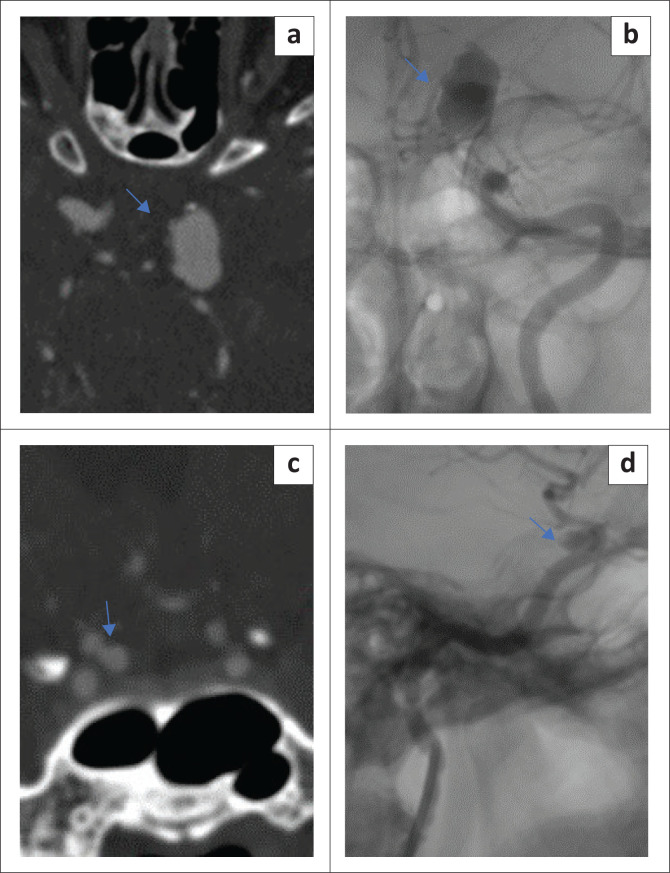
(a) CT angiography axial and (b) left internal carotid artery angiogram (non-subtracted image) frontal view showing a medium-sized (13 mm) fusiform aneurysm arising from the supraclinoid segment of left internal carotid artery (arrows) in a 65-year-old female. (c) CT angiography coronal maximum intensity projection (MIP) and (d) right internal carotid artery angiogram (non-subtracted image) lateral view showing a small (4.6 mm) saccular aneurysm arising from the medial aspect of supraclinoid segment of right internal carotid artery.
